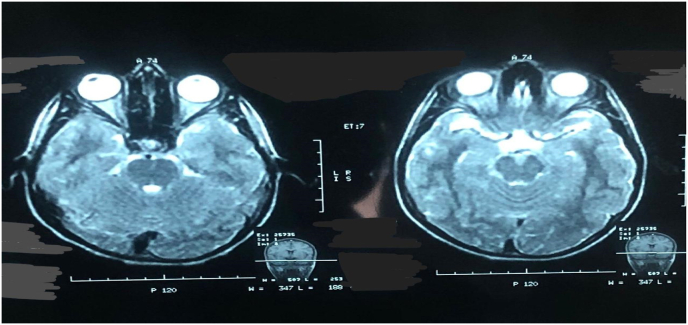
Magnetic Resonance imaging shows a normal study with no obstructive lesion in the orbit and anterior cranial fossa.
